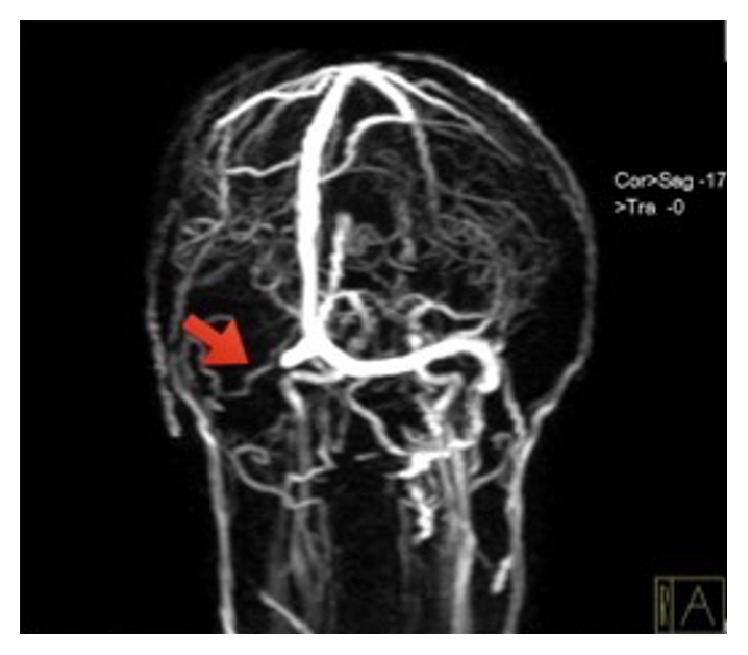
MRI venography showing right transverse and sigmoid sinus thrombosis (arrow).
